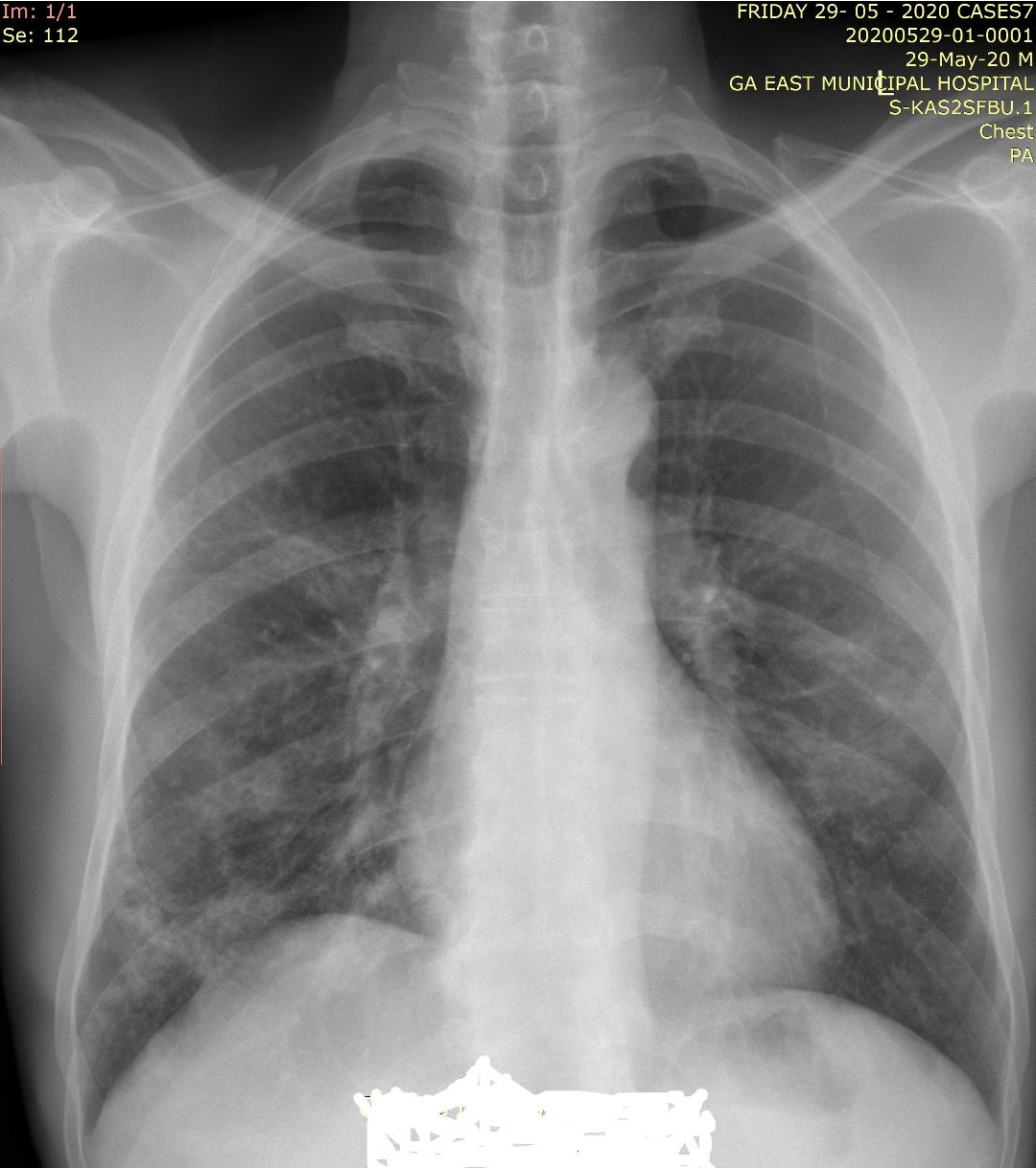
Posteroanterior chest radiograph showing patchy ground glass opacities in the right lung base and subtle ground glass opacities in both middle zones- Mild Disease
